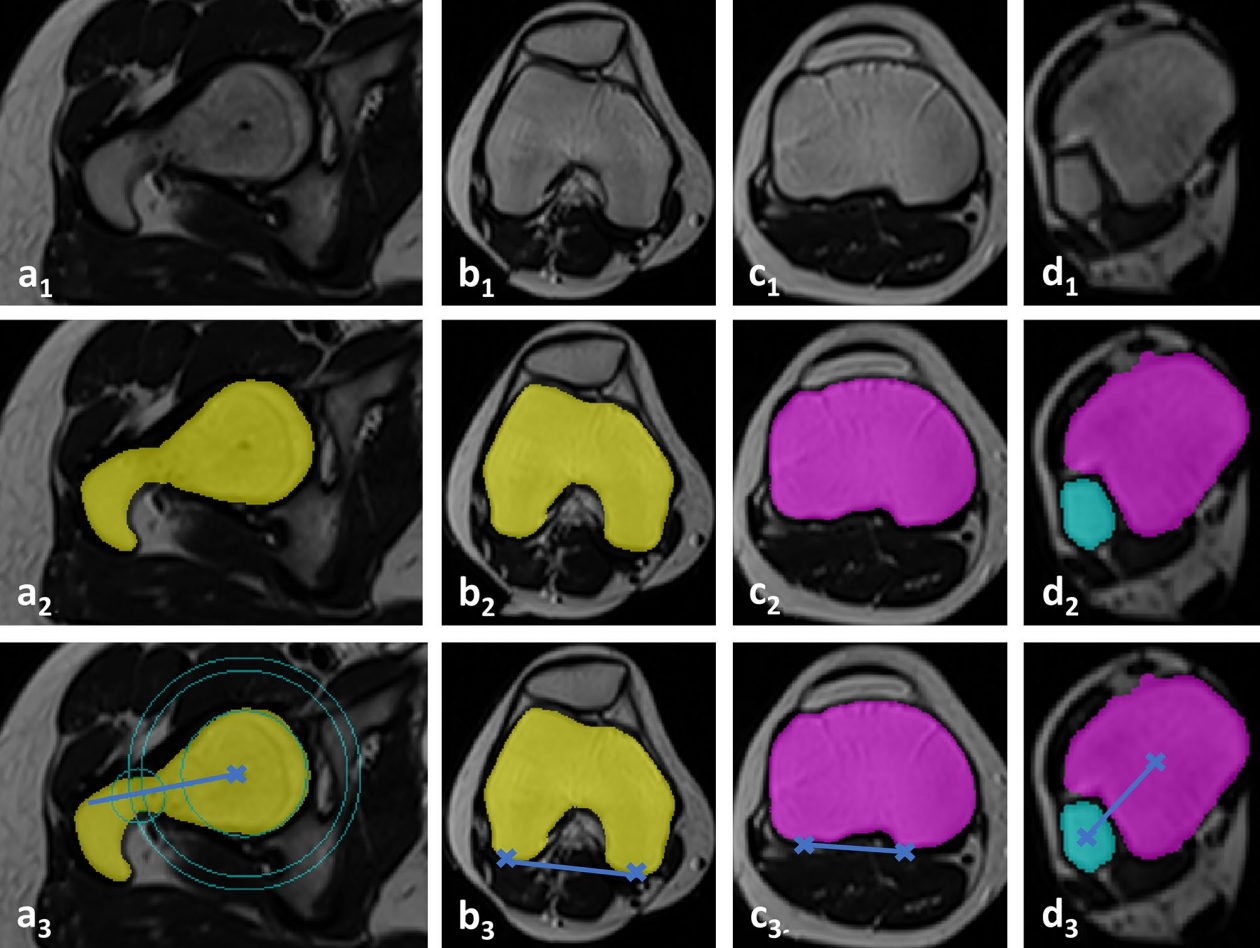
Visualization of the algorithm-based identification of the reference lines. Indicated are the original MR images (a_1_-d_1_), their segmentation outlines on selected axial images (a_2_-d_2_), and the corresponding reference lines (a_3_-d_3_) as determined by the algorithm. Proximal femur (a), distal femur (b), proximal tibia (c), and distal tibia (d). Reference lines and points are blue. For algorithmic details please refer to Fig. 4. Femur (yellow), tibia (purple), and fibula (light blue). 19-year-old female.
